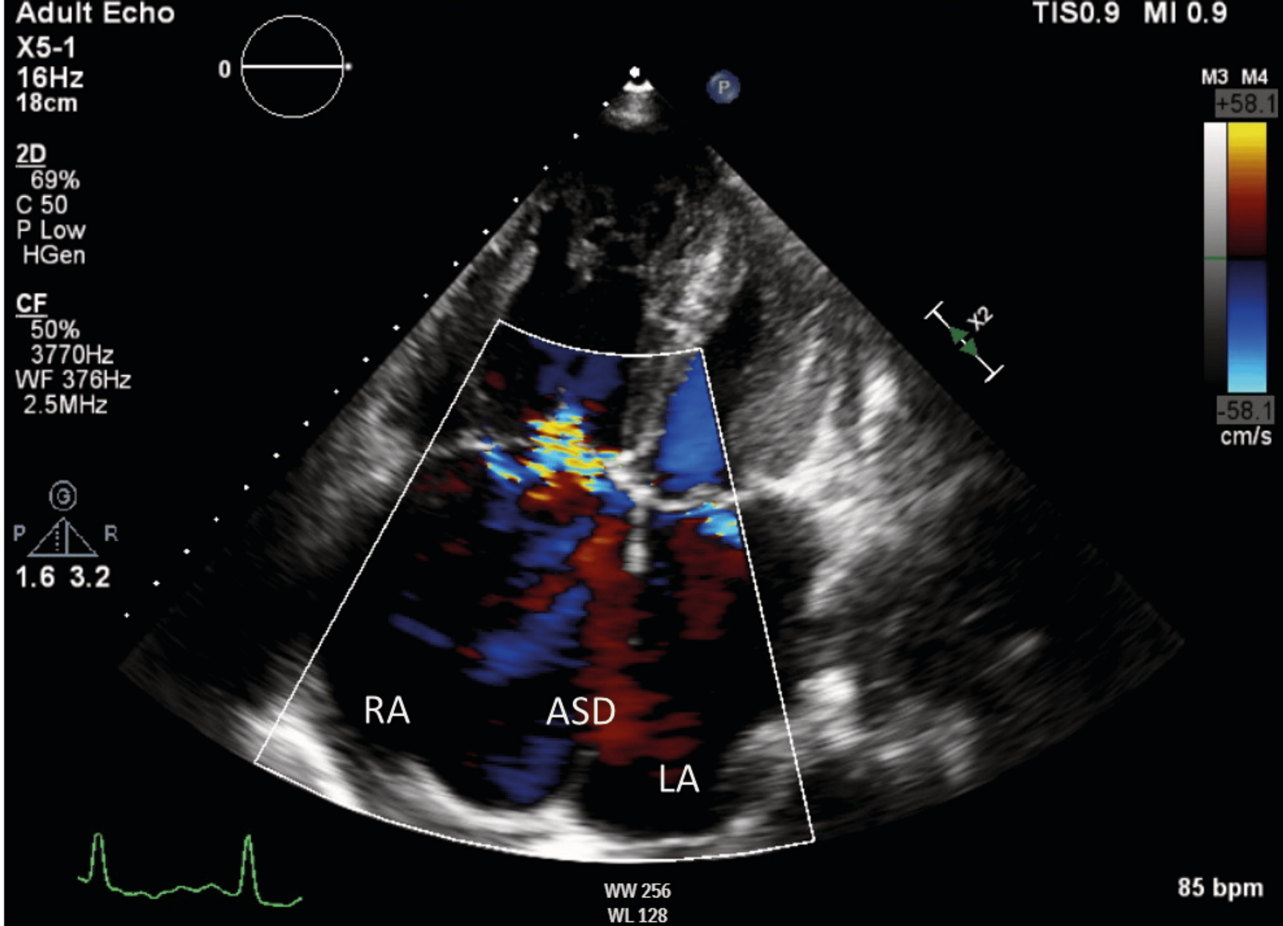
Apical four-chamber echocardiographic view The A4C echocardiogram demonstrates the presence of an ASD with a left-to-right shunt. Both the right and left atria appear dilated. ASD, atrial septal defect; LA, left atrium; RA, right atrium
